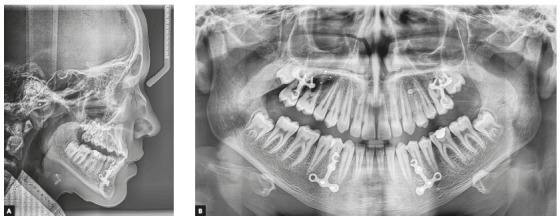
A) Post-orthopedic treatment lateral cephalometric radiograph. B) Post-orthopedic treatment panoramic radiograph.
